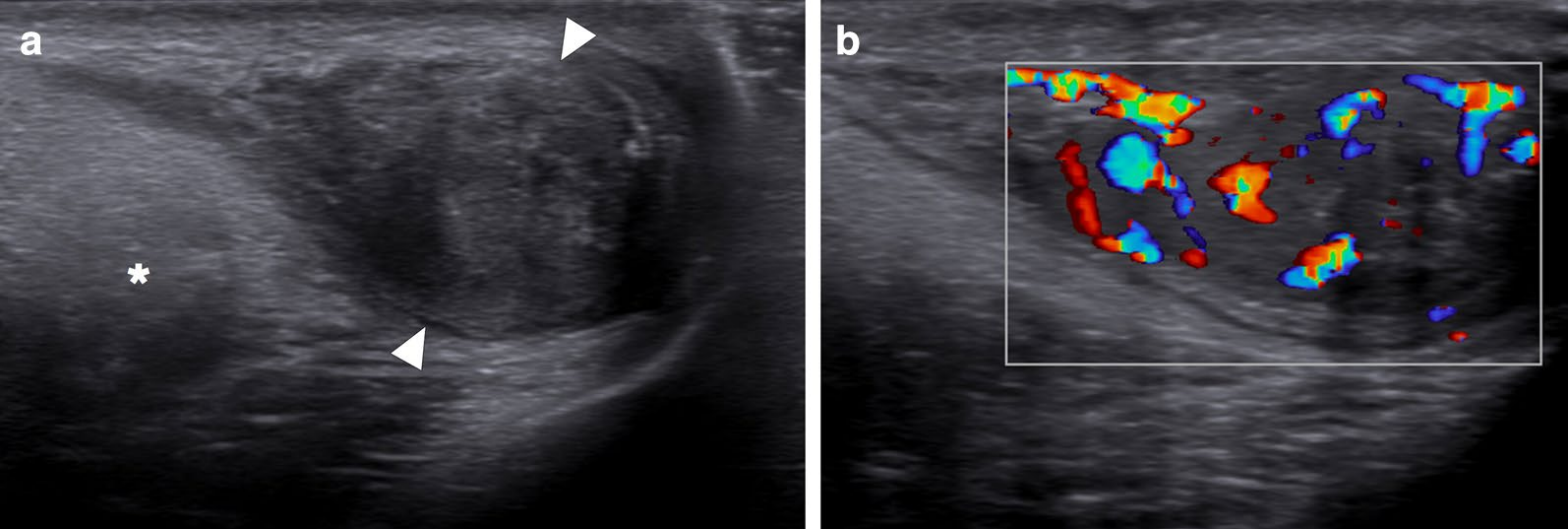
Paratesticular inflammatory pseudotumor. A 11-year-old boy who presented to ER with painless left scrotal swelling. a Gray scale US image demonstrates a heterogeneous, predominantly hypoechoic extratesticular lesion (arrowheads), adjacent to the lower pole of the left testis (asterisk). Also noted were a few scattered intralesional calcifications. b Color Doppler US exam shows widespread internal vascularity. The findings suggest an intrascrotal-extratesticular tumor, such as rhabdomyosarcoma. Surgical removal of the tumor, with preservation of the left testis, instead found paratesticular inflammatory pseudotumor
